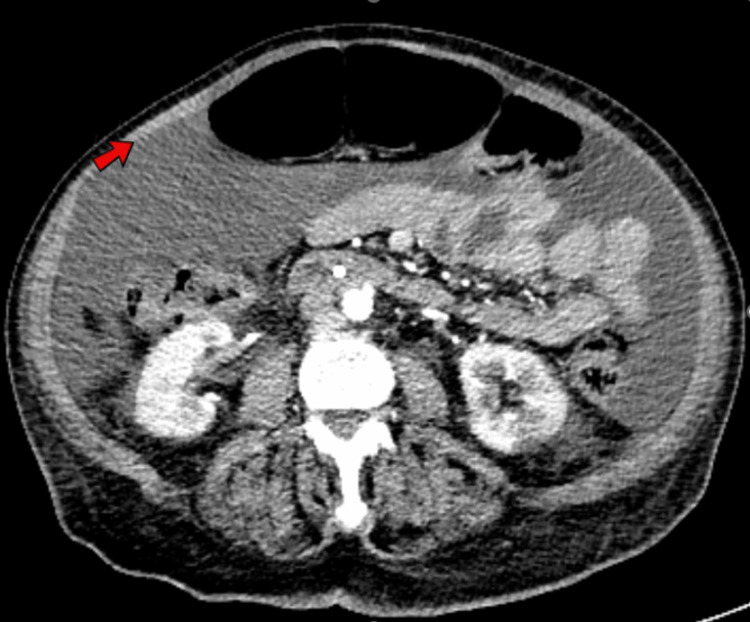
A CT scan of the chest, abdomen, and pelvis showed moderate ascites in the peritoneal cavities (red arrow) and borderline enlarged mesenteric and para-aortic lymph nodes.
